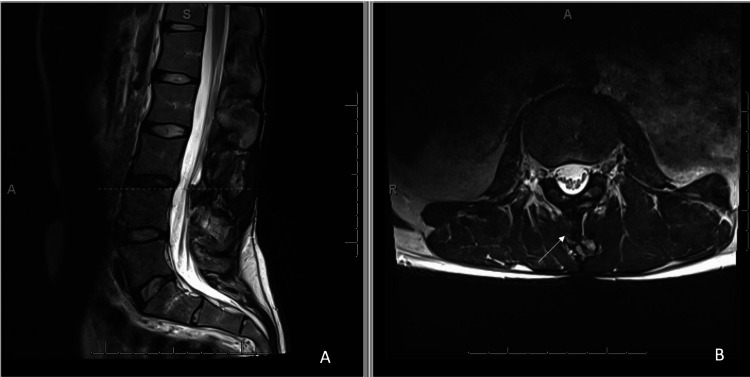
MRI lumbar spine without contrast, sagittal T2 and axial T2 at L3-L4 level (A) L3-L4 post-laminectomy changes with disc desiccation at the L3-L4 vertebral body level (dashed line); (B) Cross-section of post-laminectomy site with calcifications in and around the interlaminar space (arrow).
